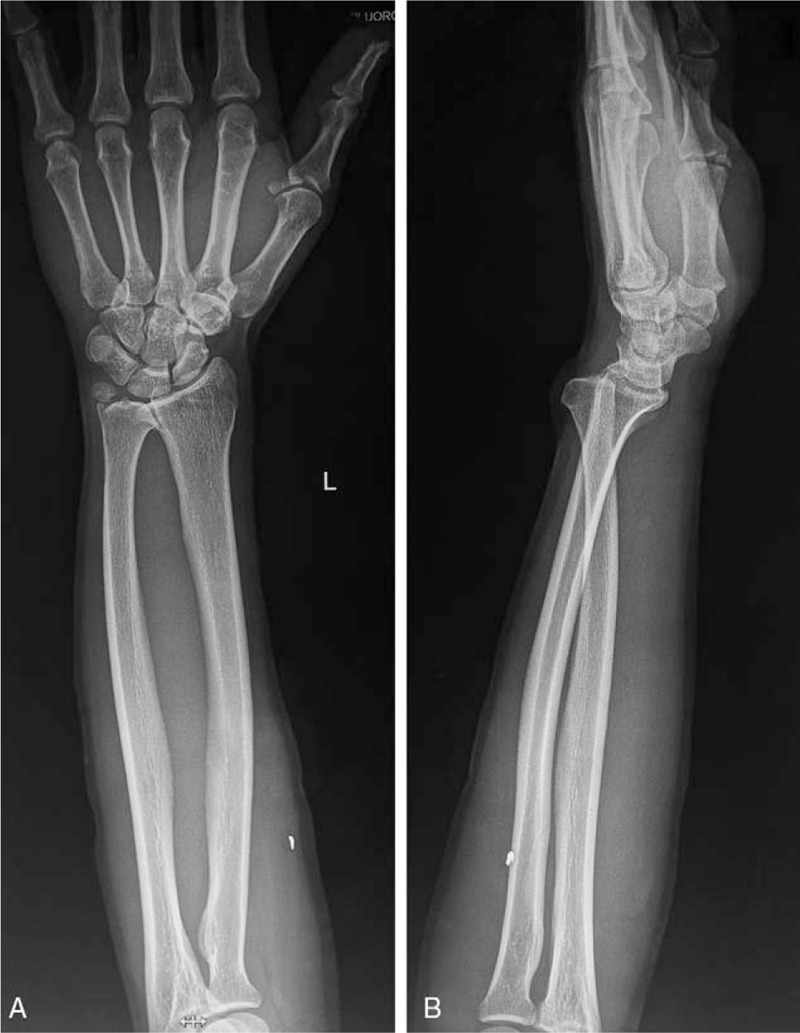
X-ray films of the foreign body. There was a 3-mm high-density shadow in the superficial soft tissue. Additionally, an old fracture of the left ulnar styloid process was found.
